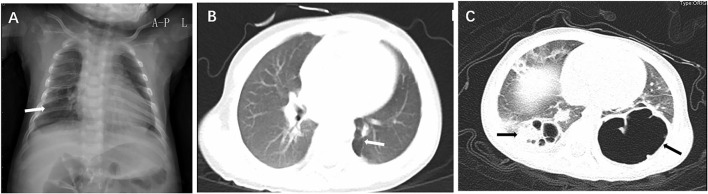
Chest X-ray showed translucency of the right lower lobe (A, arrow); CT scan of the chest showed a single cyst at the left lower lobe (B, arrow) and bilateral cysts in left and right lower lobes (C, arrows).
